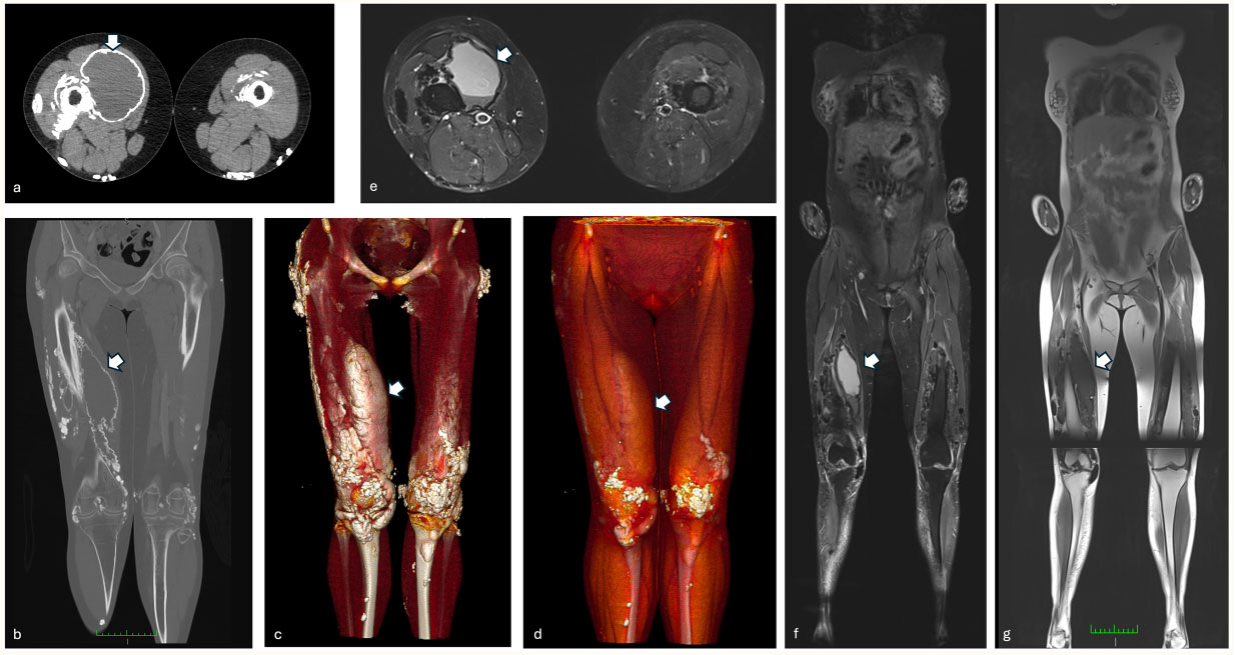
CT and MR features of milk of calcium calcinosis in scleromyositis. Leg axial CT (a) of a 15-year-old female with scleromyositis showing a fluid collection with a calcific rim in the right vastus medialis, corresponding to the clinically reported slow-growing mobile lump. Coronal (b) and 3D (c and d ) reconstructions show the extent and the calcific rim of the milk of calcium lesion. Axial short inversion time inversion recovery (STIR) (e), coronal STIR (f ) and T1-weighted (g ) whole-body MR, performed 8 years later, nicely demonstrating the fluid content and the peripheral calcific margins of the lesion that remained stable over time. Multiple soft tissue calcifications are visible on CT (as hyperdense areas) and MR (as signal voids) as part of the calcinosis pattern which increased over time
